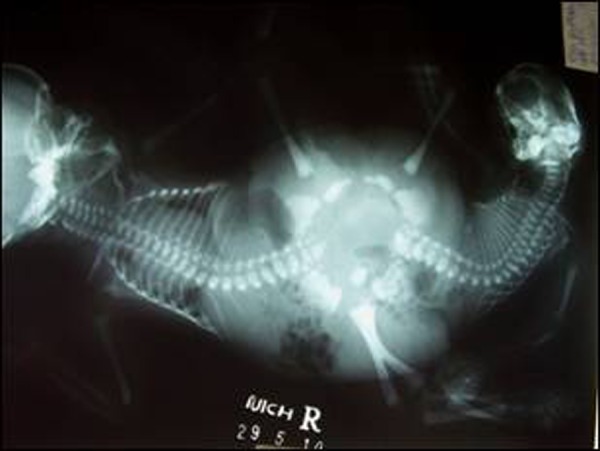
Figure 3: X-ray babygram of the conjoined twins
